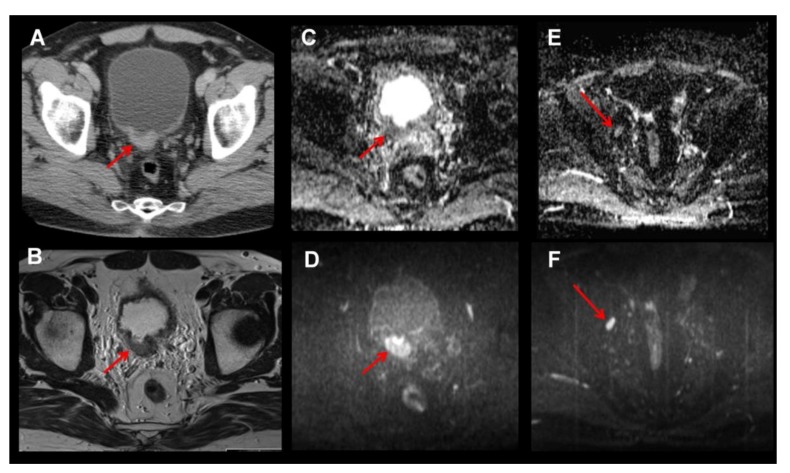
Bladder tumor at dorsal bladder wall visualized by CT as a wall thickening with contrast enhancement (A). Of note, soft tissue contrast is superior in MRI compared to CT (B, T2w axial). Additional diffusion weighted imaging (DWI; C–F) improves delineation of the tumor mass as a hyperintense mass in the b-value images (D, b800 image) and allows evaluating apparent diffusion coefficient (ADC) values (C) as potential marker of tumor cellularity. Moreover, DWI might be helpful for evaluating lymph node metastasis, as metastatic lymph nodes usually also show lower ADC values (F, b800 image showing a hyperintense iliacal lymph node on the right; E, low ADC value suggests malignancy).
